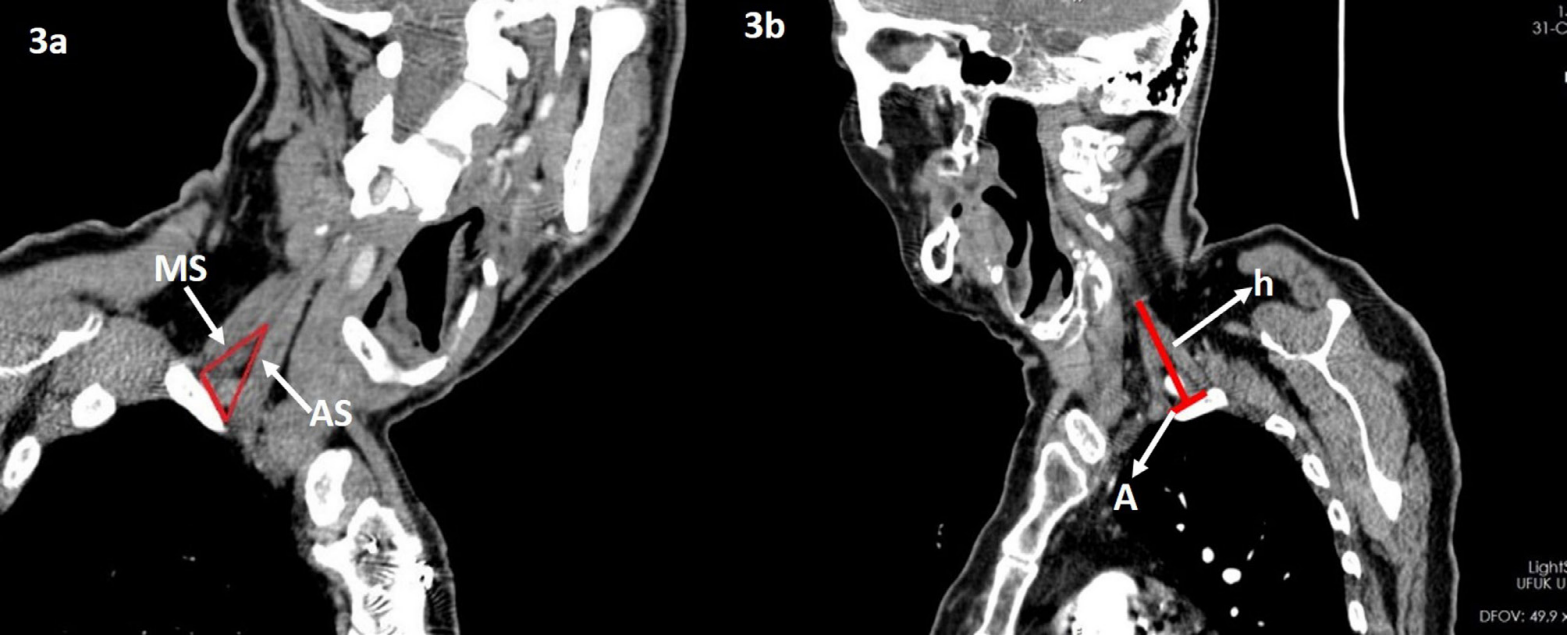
Thoracic CT sections of patients who were operated with the diagnosis of TOS. MS: Middle scalene, AS: Anterior scalene, h: Interscalene triangle height, A: Interscalene triangle base length.
